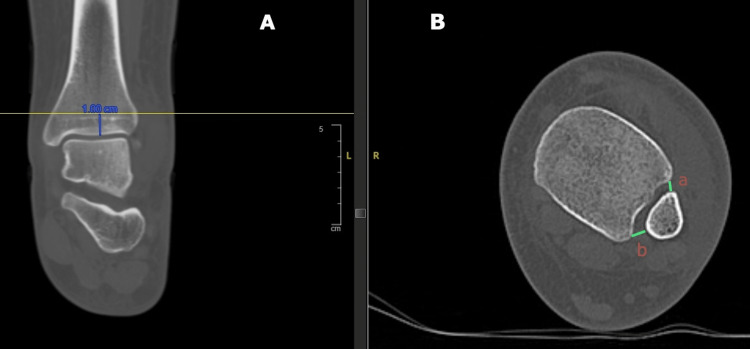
Image A is a coronal CT scan image with the corresponding axial CT scan in image B showing measurements for the distal tibiofibular syndesmosis at a level 1 cm above the tibial plafond a: anterior interval; b: posterior interval
